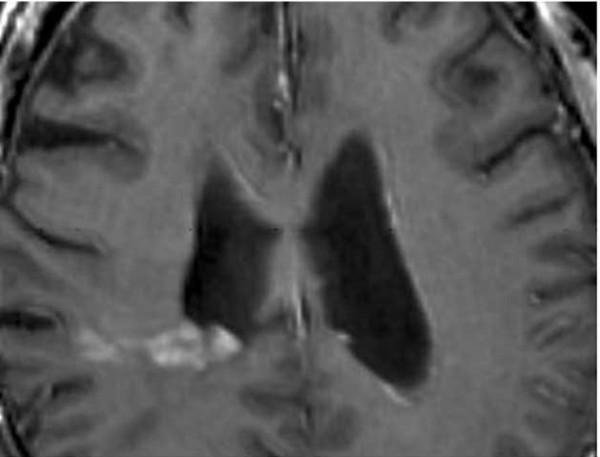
Close up of an MRI showing enhancement along the right lateral ventricle.
